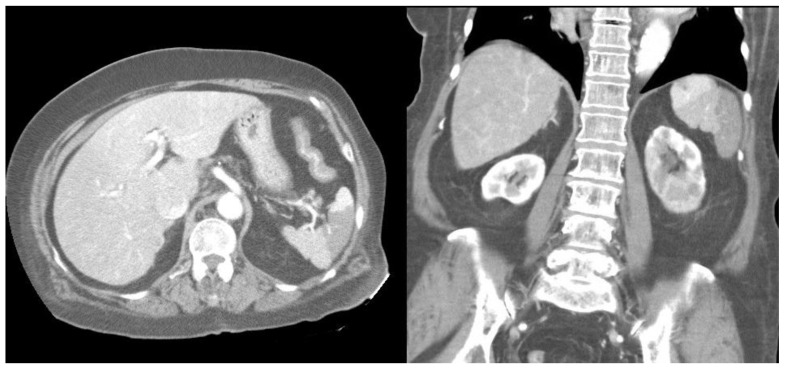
Concurrent splenic and left renal infarction. Contrast-enhanced abdominal CT images showing wedge-shaped hypodense regions within the spleen on the axial view (left panel) and within both the spleen and the left kidney on the coronal view (right panel).
